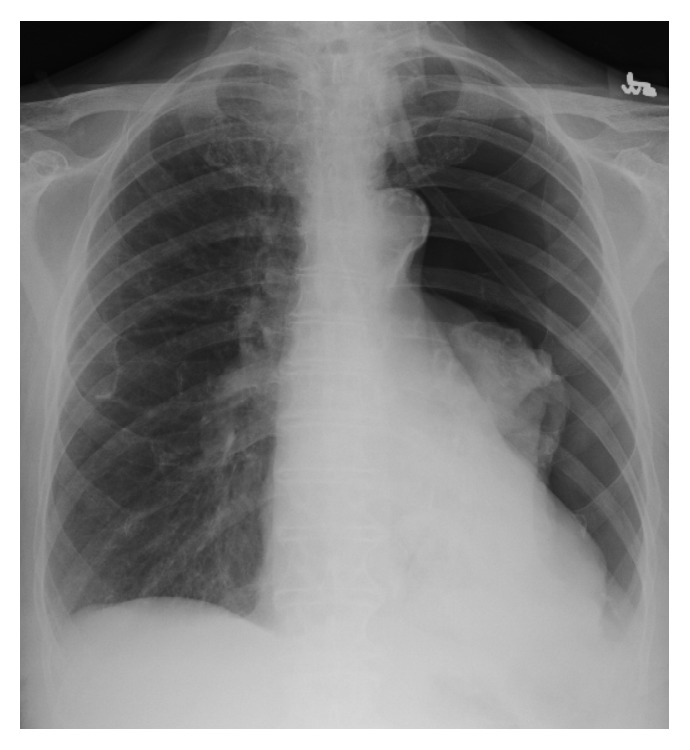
Initial portable chest X-ray showing significant left lung collapse.
